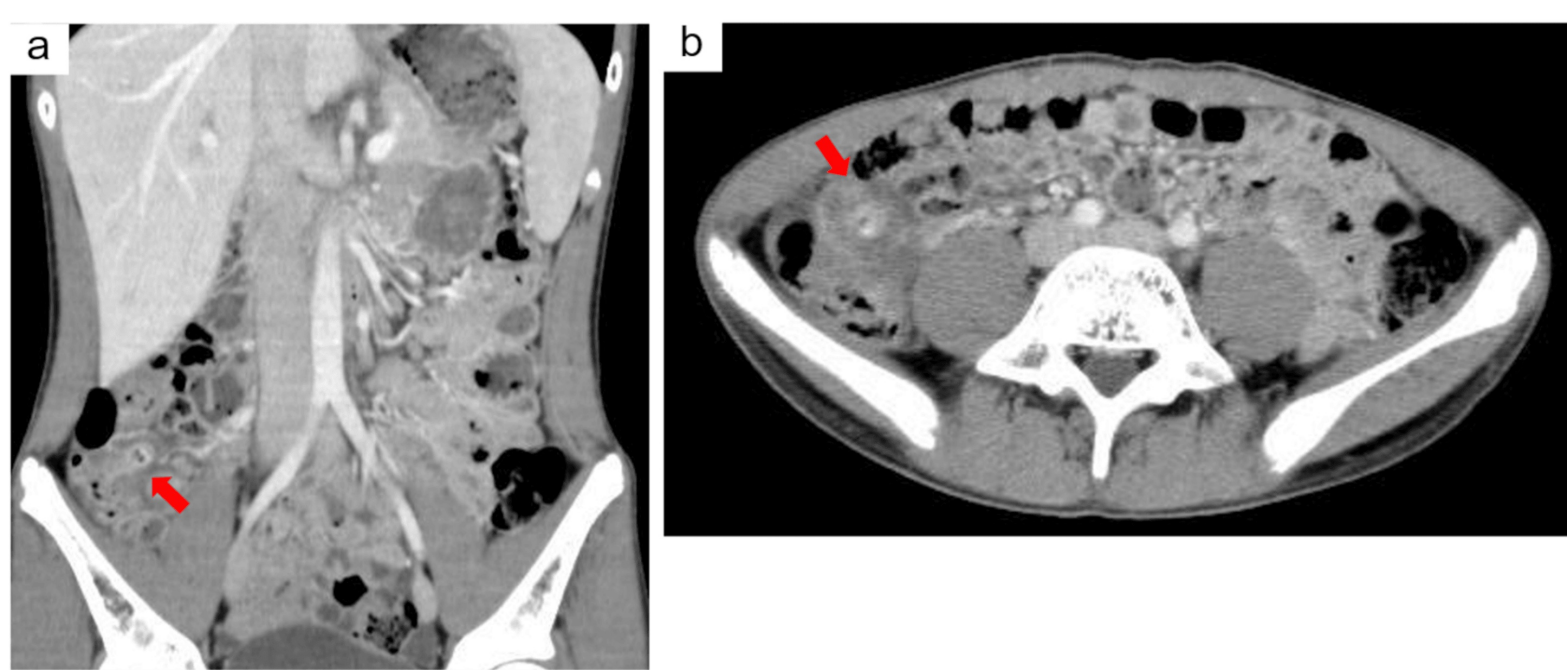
Contrast-enhanced abdominal computed tomography images (a) Coronal section: A solitary diverticulum was observed in the ascending colon, accompanied by wall thickening and increased density of the surrounding adipose tissue (arrow). (b) Axial section: A diverticulum with wall thickening and pericolic fat stranding (arrow).
